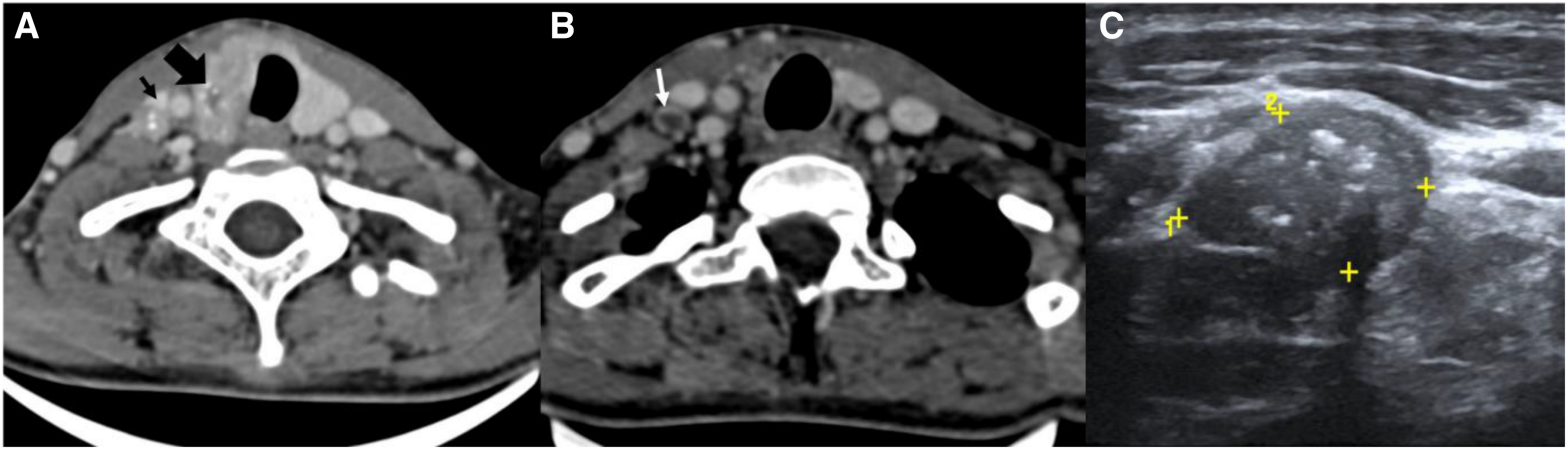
CT scan (A, B) and USG images (C) of the neck of a known case of papillary Ca thyroid. (A) CT scan images showing a heterogeneously enhancing thyroid mass (thick black arrow) with microcalcifications within and a similar morphology neck node at the right level IV (thin black arrow). (B) CT scan image of the same patient at a lower level showing a peripherally enhancing node with central necrosis (white arrow). (C) USG image of the same patient showing a metastatic node at the right level IV with microcalcifications within.
